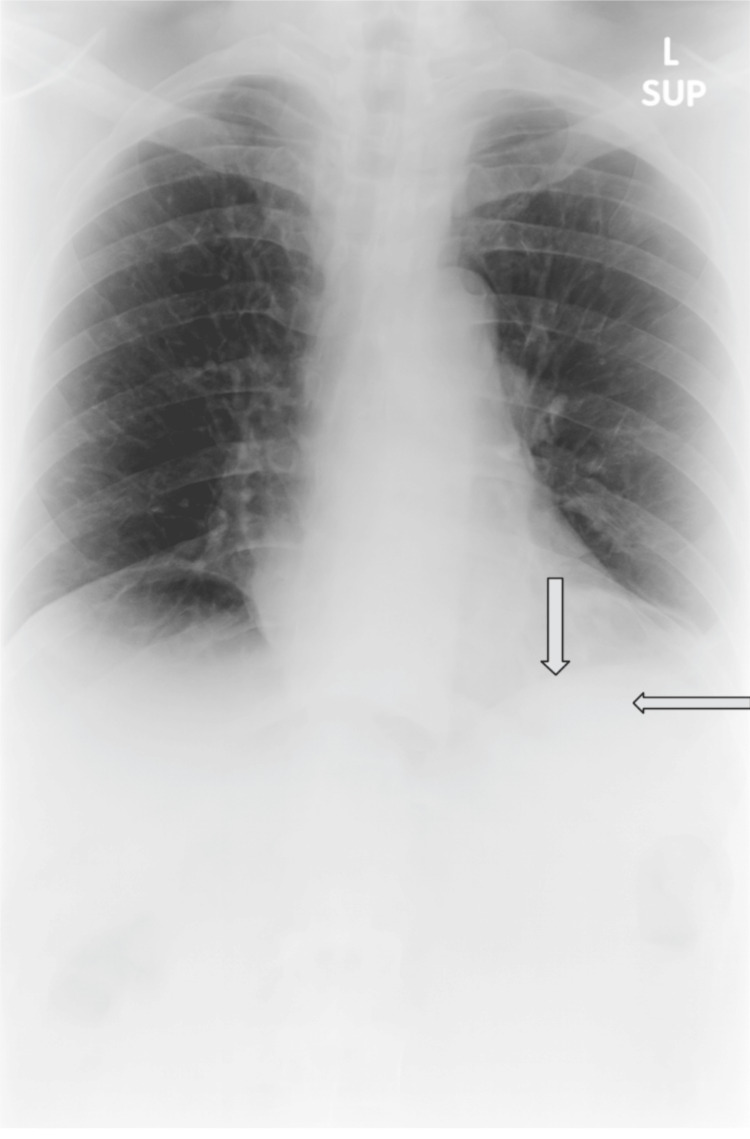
Chest X-ray of the patient revealing signs of consolidation of the left lower lobe, consistent with lobar pneumonia
